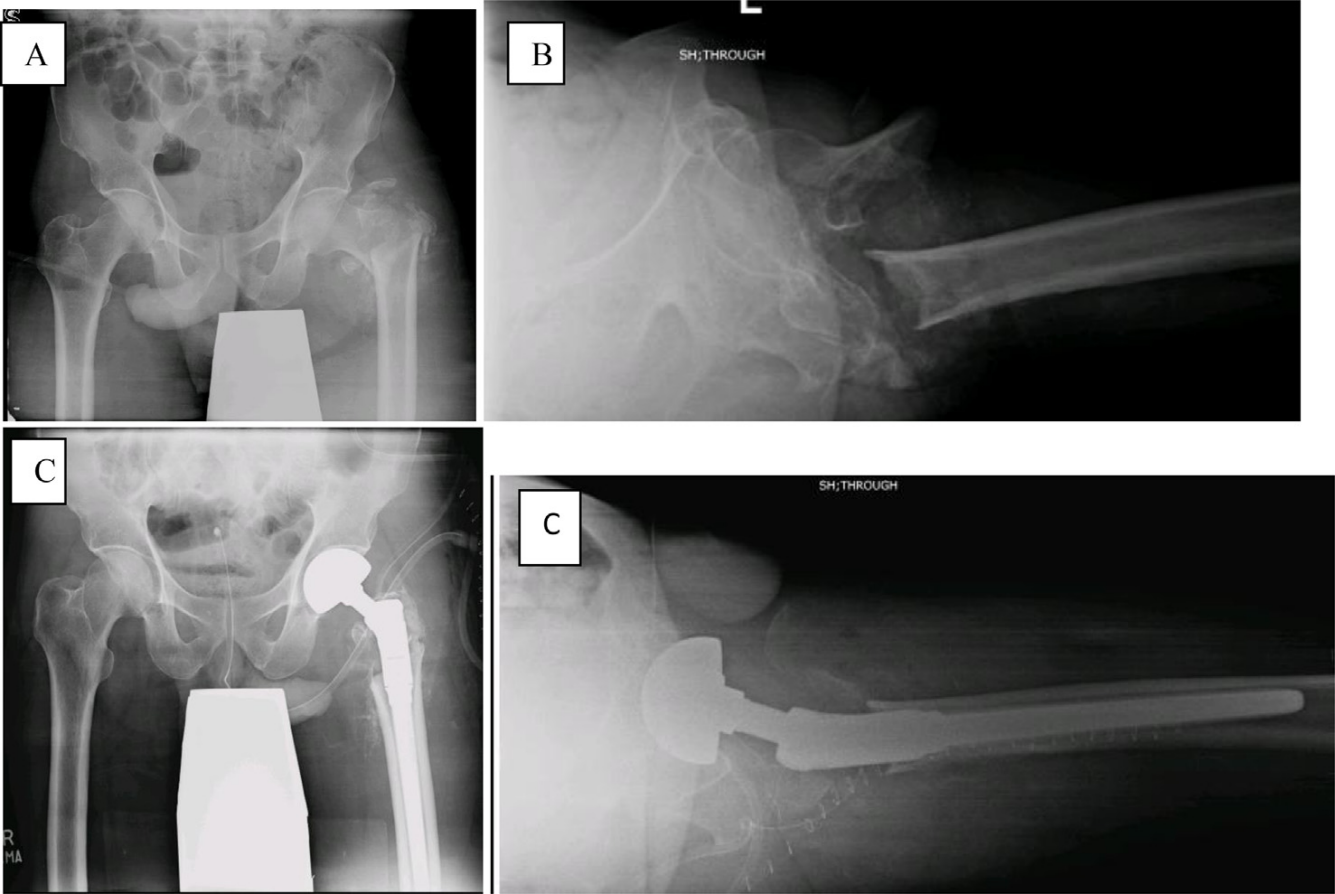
Pre-operative x-rays pelvis AP [A] and left hip lateral shoot through [B] Showing intertrochanteric fracture. Post-operative x-rays pelvis AP [C] and left hip lateral shoot through [D] showing proximal femur replacement.
